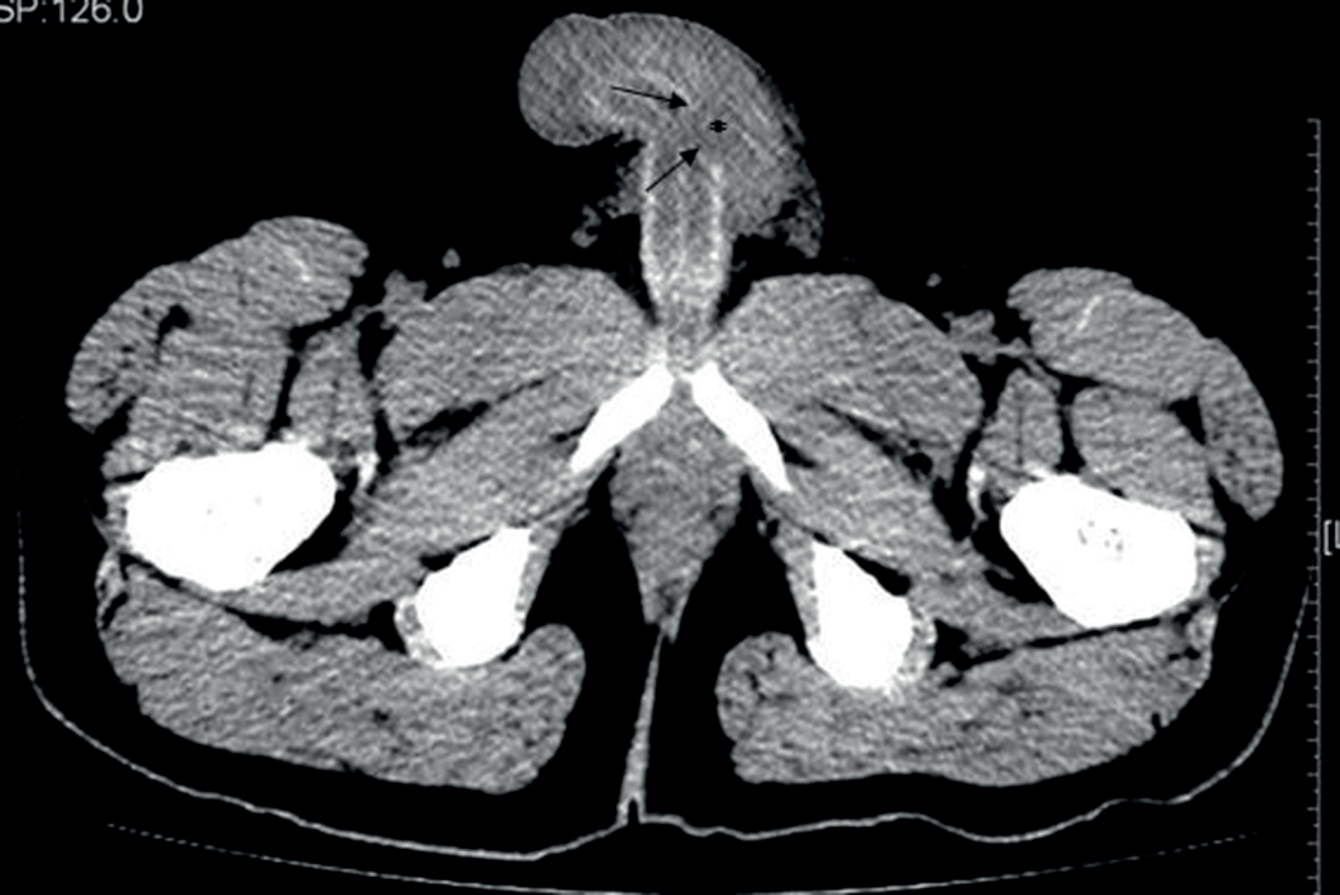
Twenty-eight - old man with penile fracture. Axial CT image obviously demonstrated unilateral 0.8cm x 1.4cm area of tear of the left lateral tunia albuginea with adjacent hematoma. Arrows indicate the free edges of tunica defect and asterisks show the associated hematoma.
